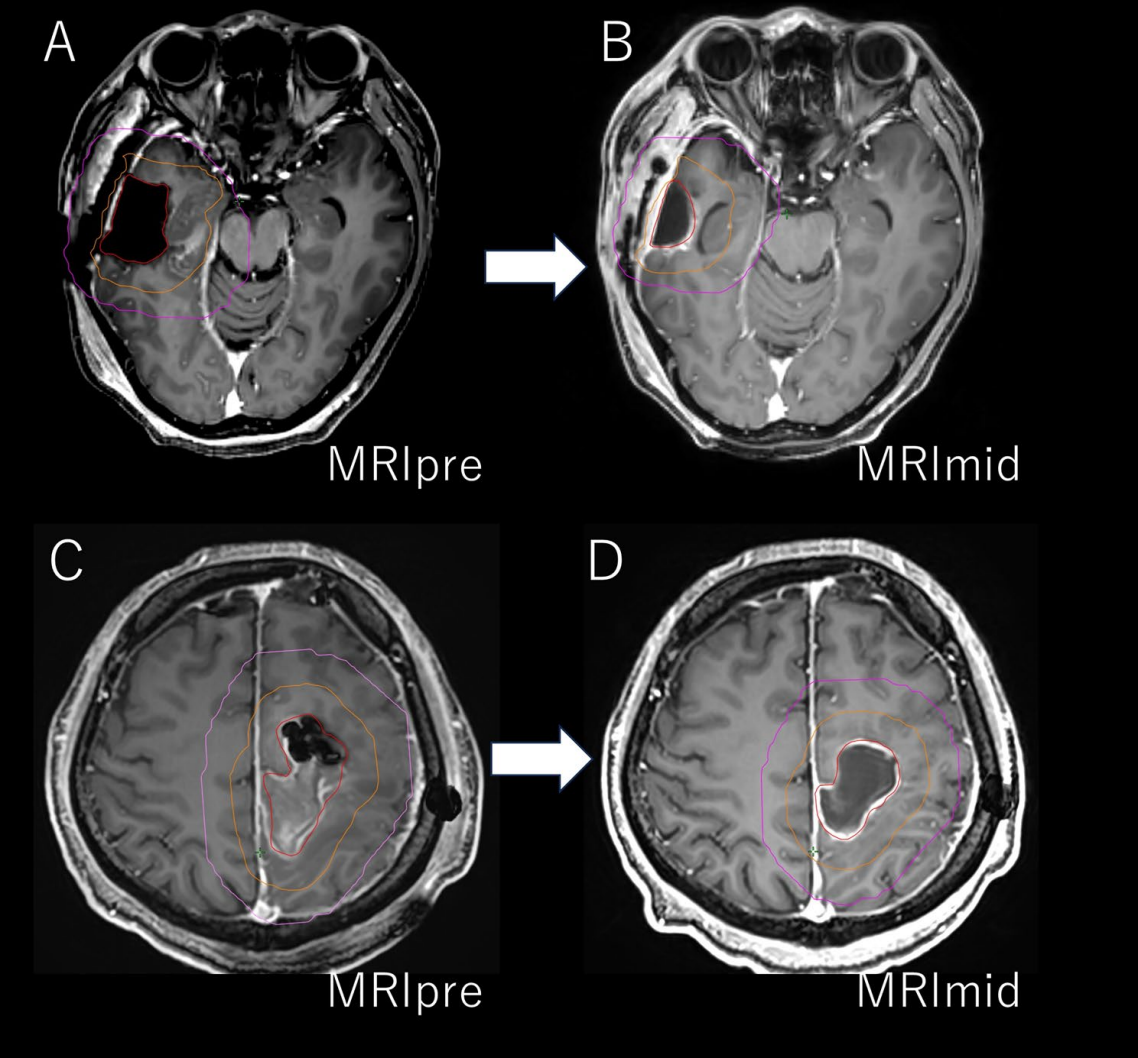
Representative examples of single-time-point offline adaptive boost planning. A–B: 42-year-old man, right temporal lobe glioblastoma. A: Baseline contrast-enhanced T1-weighted MRI (MRIpre) with resection cavity (red) and CTV (orange) contours and the corresponding 95% isodose line (magenta). B: Mid-treatment MRI (MRImid) showing resection-cavity (red) shrinkage and the adapted CTV (orange); the adapted 95% isodose line (magenta) encloses a 28% smaller volume than MRIpre. C–D: 53-year-old man, left parietal lobe glioblastoma. C: MRIpre with resection cavity (red) and CTV (orange) contours and the corresponding 95% isodose line (magenta). D: MRImid shows resection-cavity (red) shrinkage and the adapted CTV (orange); the adapted 95% isodose line (magenta) encloses a 40% smaller volume than MRIpre
